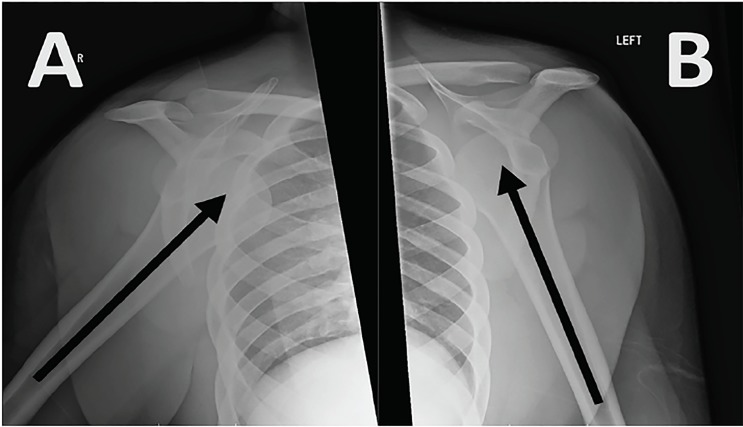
Findings on arrival. (A) Shoulder radiograph showing anterior dislocation of the right shoulder joint (arrow). (B) Shoulder radiograph showing anterior dislocation of the left shoulder joint (arrow).
